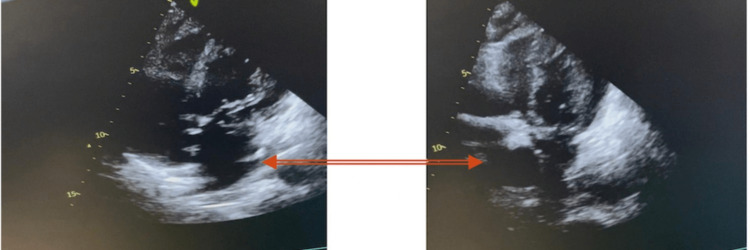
Post-surgical echocardiography Post-surgical images show a decrease in global contractility, apical-septal and apical-lateral wall akinesia/hypokinesia, and moderate mitral valve regurgitation. Images show the difference between diastole (left) and systole (right).
